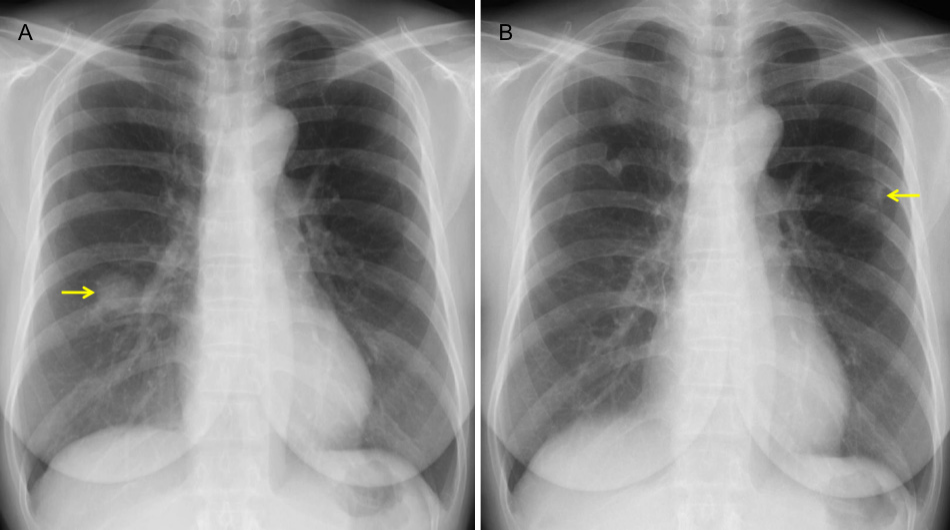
Chest X-rays taken before the first (A) and second (B) operation. (A) Chest X-ray shows an ill-defined consolidative lesion in the right middle lung field. (B) Chest X-ray shows an ill-defined consolidative lesion in the left middle lung field. The arrows indicate the lesions.
